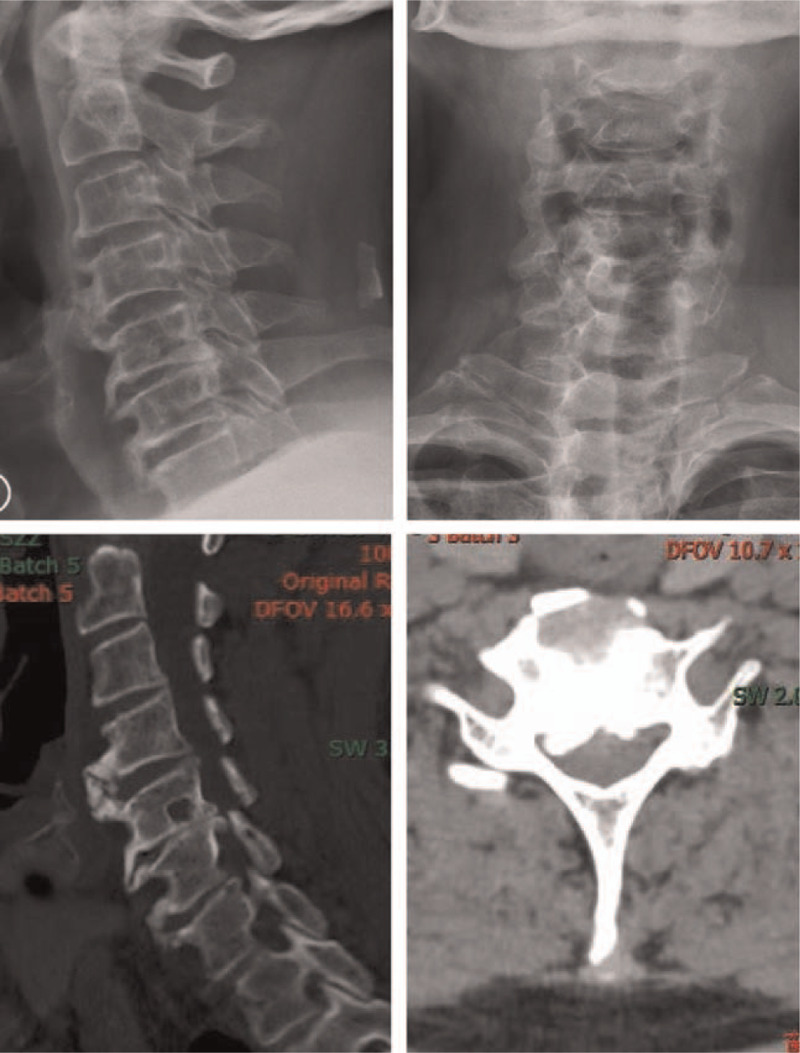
Preoperative imaging of the cervical spine. A and B, The X-ray examination showed C4-7 anterior vertebral osteophyte, nuchal ligament calcification, and the intervertebral space of C5/6 was narrowed. C and D, Axial and sagittal CT showed C4-7 anterior vertebral osteophyte and a calcification in the C5/6 spinal canal.
